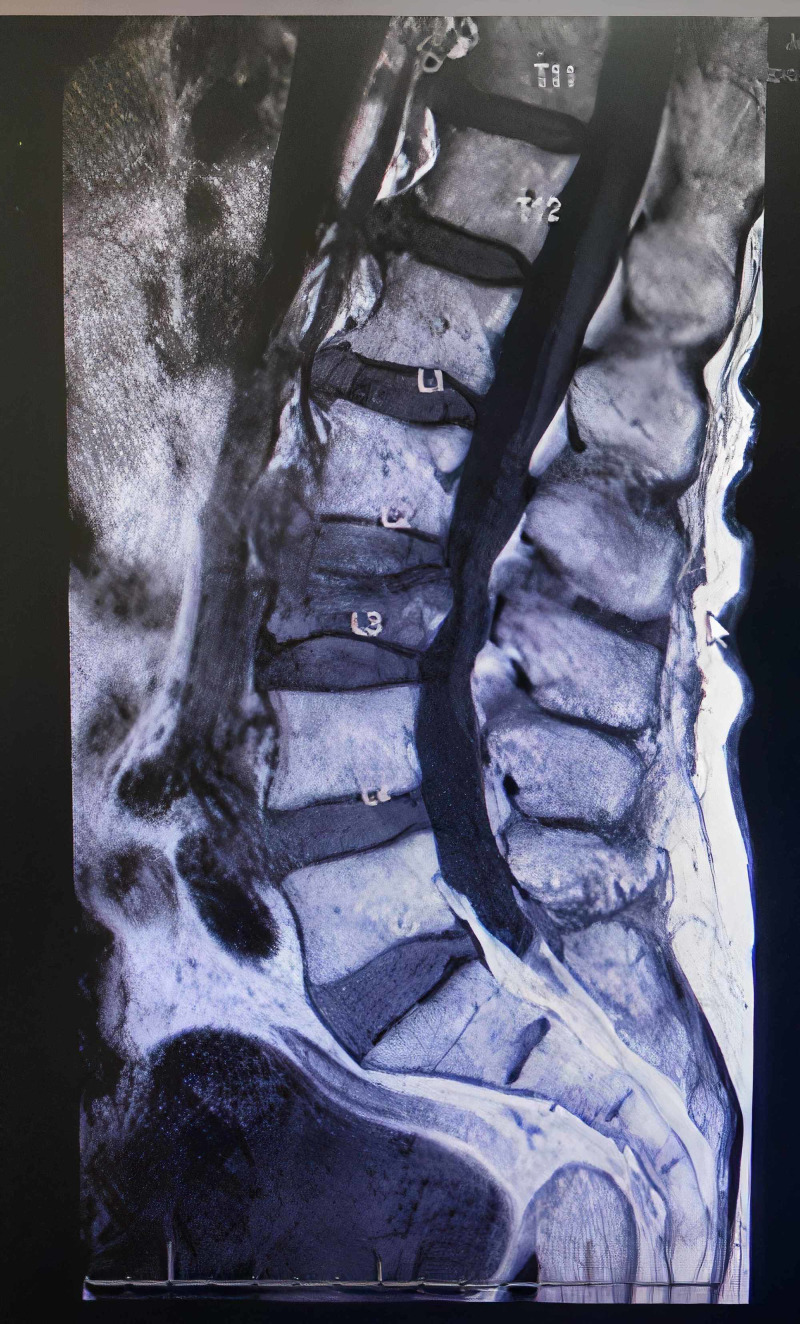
Preoperative MRI, sagittal plane, showing subacute L3 compression fracture with a 50%-60% loss of height
